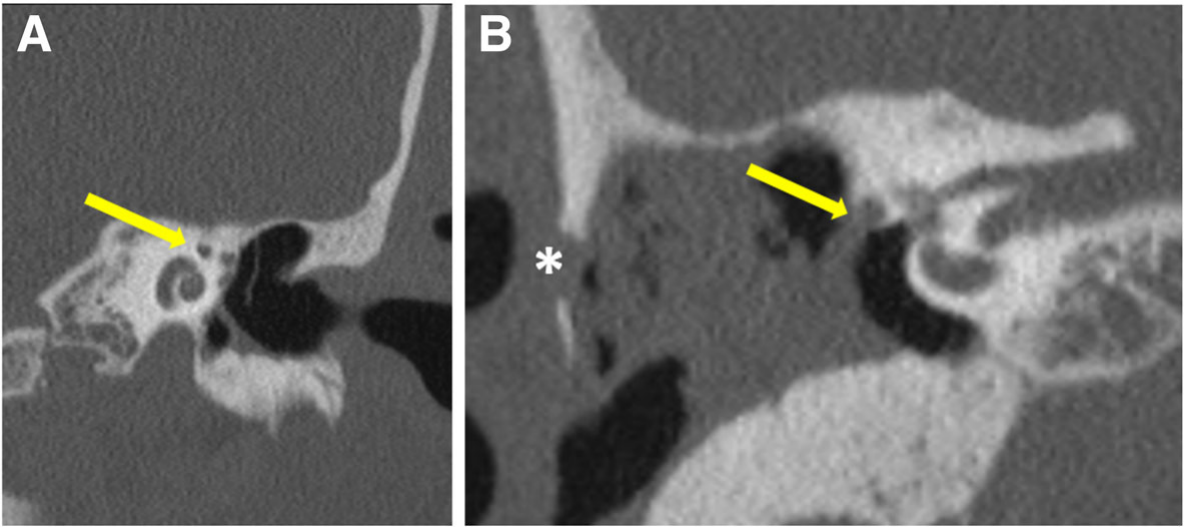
Coronal (a) CT image reveals the “snail eye” view where the turns of cochlea form the body of snail and labyrinthine and tympanic segments of facial nerve being the eyes (arrow). Coronal (b) CT image shows the erosion of the floor of the horizontal segment of the facial canal (arrow). Erosion of lateral mastoid cortex is also seen (asterisk)
